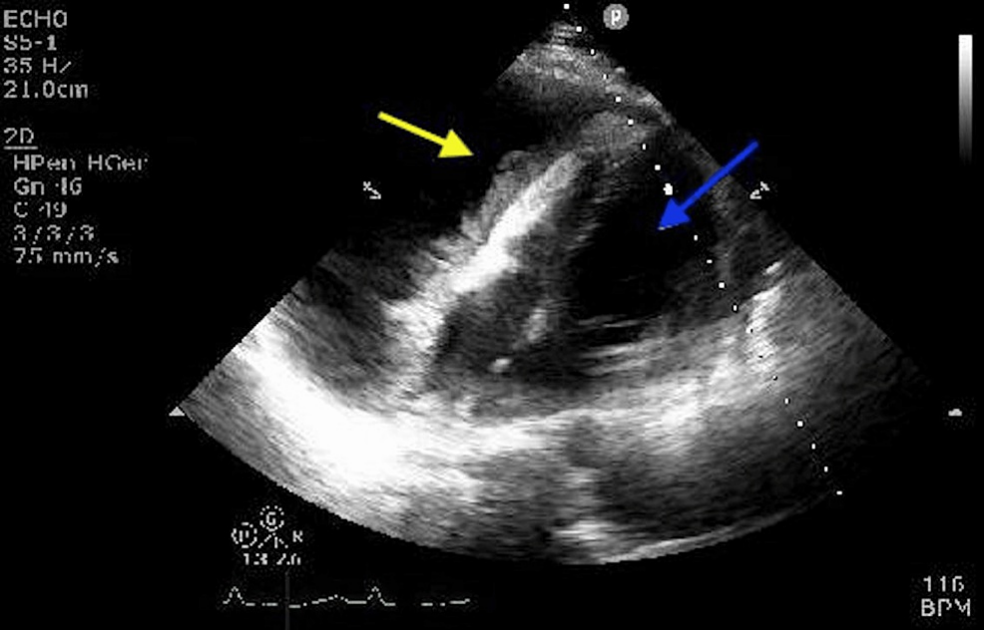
Echocardiogram image showing large pericardial effusion. Yellow arrow pointing to pericardial effusion with threads. Blue arrow pointing to left ventricle.
